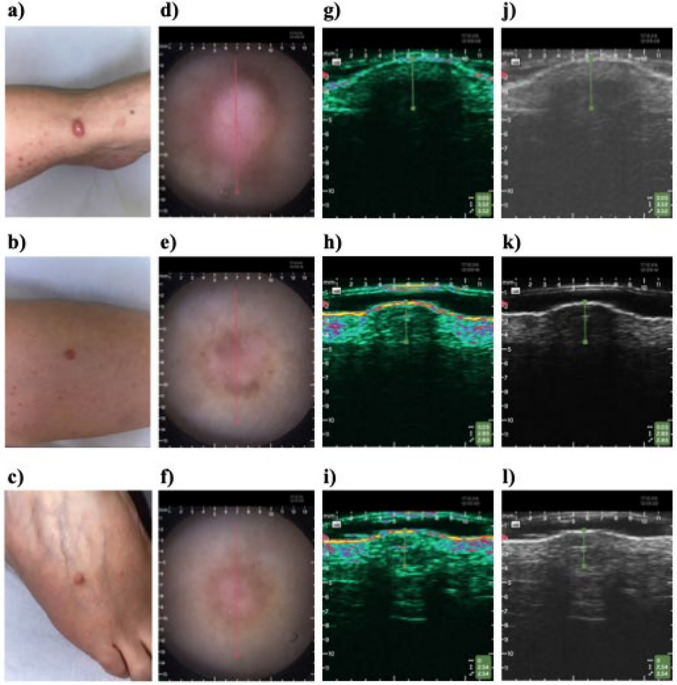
Dermatofibromas in a 70-year-old female patient, Fitzpatrick skin type II. a–c Clinical images showing oval (a) or round (b, c) pigmented, raised lesions on the lower extremity. d–f Dermoscopic image showing a skin-coloured central area with irregular peripheral pigmentation composed of brown and red structures. g–l Dermoscopy-guided high-frequency ultrasound (DG-HFUS) images demonstrating predominantly hypoechoic dermal lesions with depths ranging from 2.8 to 3.5 mm with small internal hyperechoic areas, have poorly defined margins, and show protruding hypoechoic bands extending into the surrounding dermis, occasionally reaching the hypodermis
